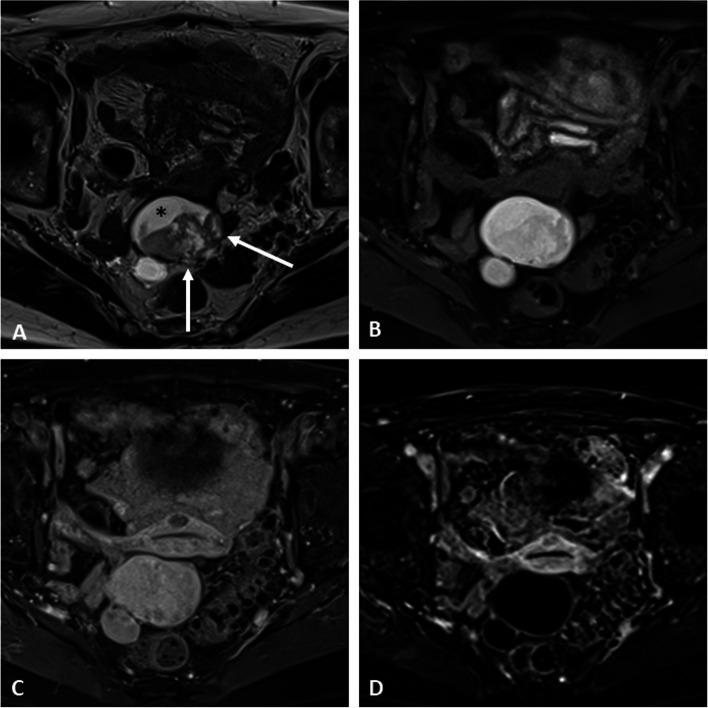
Differential diagnosis: right ovarian endometrioma in an 84-year-old woman with a history of breast cancer. Axial T2-weighted image (a) shows a right ovarian mixed lesion containing simple fluid (asterisk) and solid components (arrows). Due to the hemorrhagic filling, potential enhancement of the solid portion is difficult to appreciate between unenhanced (b) and enhanced (c) fat-suppressed T1 images, whereas the subtraction sequence perfectly displays the lack of enhancement of the solid component (d). The patient underwent surgery: pathology analysis confirmed the presence of blood clots within a benign endometrioma
